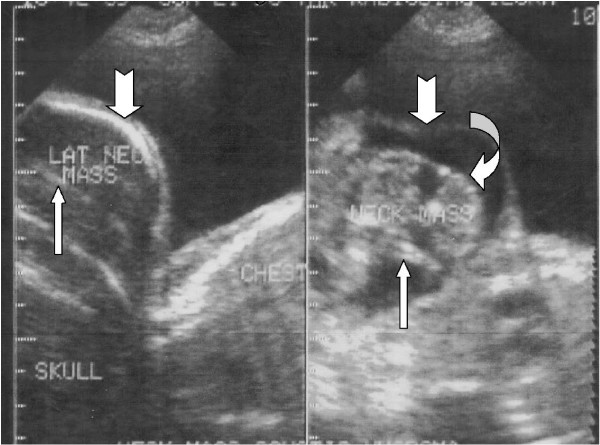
Sonograms of the foetal neck mass, midline longitudinal scan (left image) and left paramedian scan (right image), showing a large cyst that resembles a gestational sac (notched arrow). Note the central irregular solid tissue containing long bone (white arrow) and vertebral bone (curved arrow).
